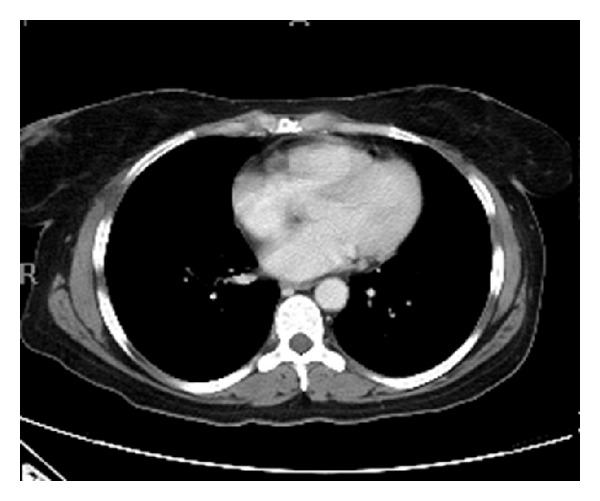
CT of abdomen in 46-year-old female with acute onset of abdominal pain with radiation of pain to back and history of pancreatitis.
